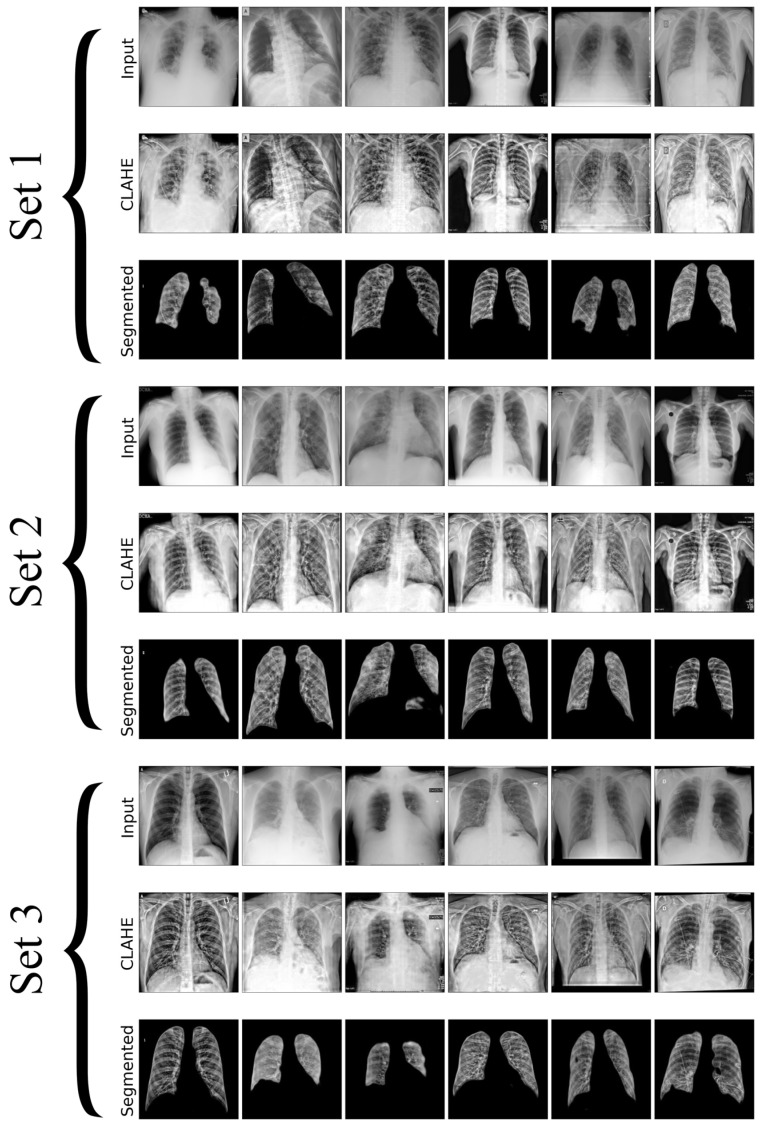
Side-by-side comparison of a chest X-ray before CLAHE enhancement, after CLAHE enhancement, and segmented images.
